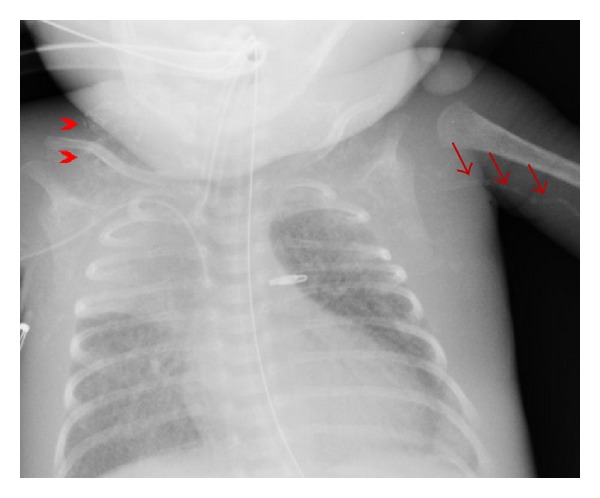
Chest X-ray shows chest soft tissue calcification (arrow heads) and calcification along the left upper arm blood vessels (arrows).
